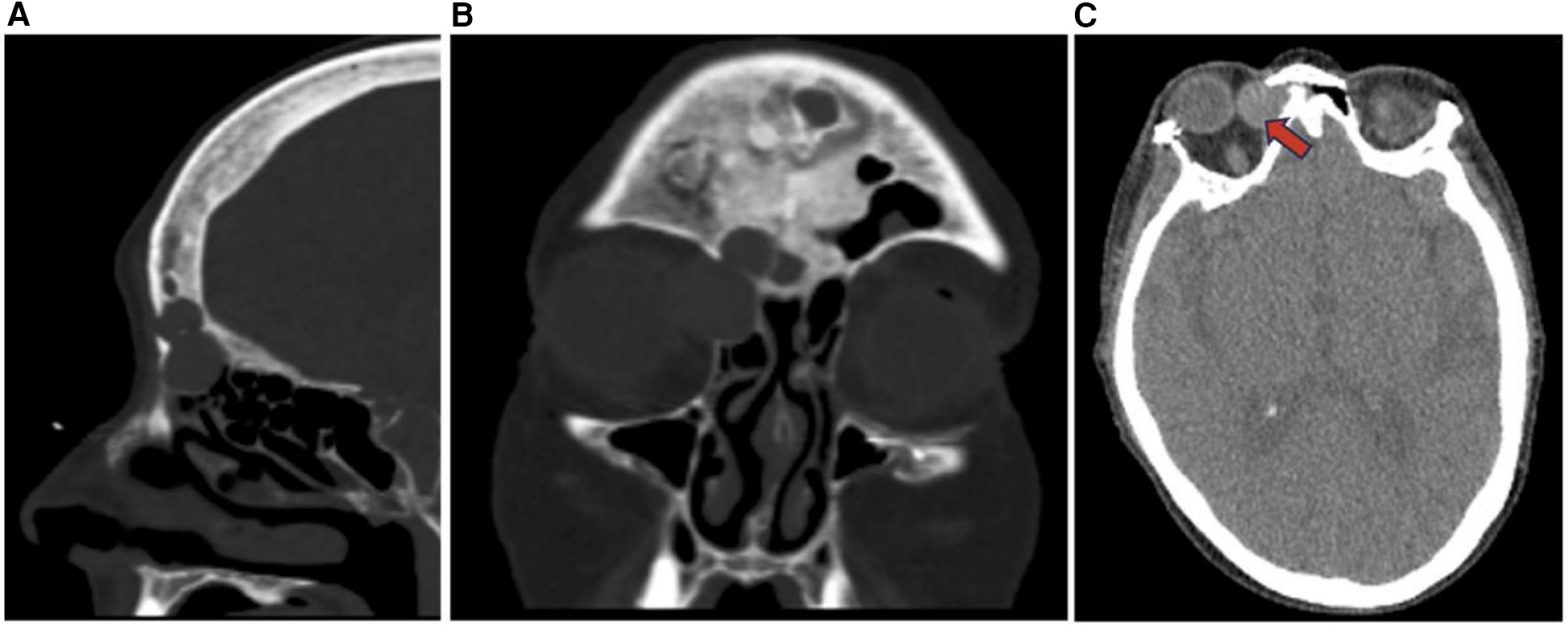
Pleomorphic adenoma sagittal (A), coronal (B), and axial (C) CT showing non-destructive expansile soft tissue lesions with cortical thinning in the right paranasal sinuses (red arrow) including the ethmoid sinus and frontal sinus possibly representing a neoplasm such as pleomorphic adenoma.
